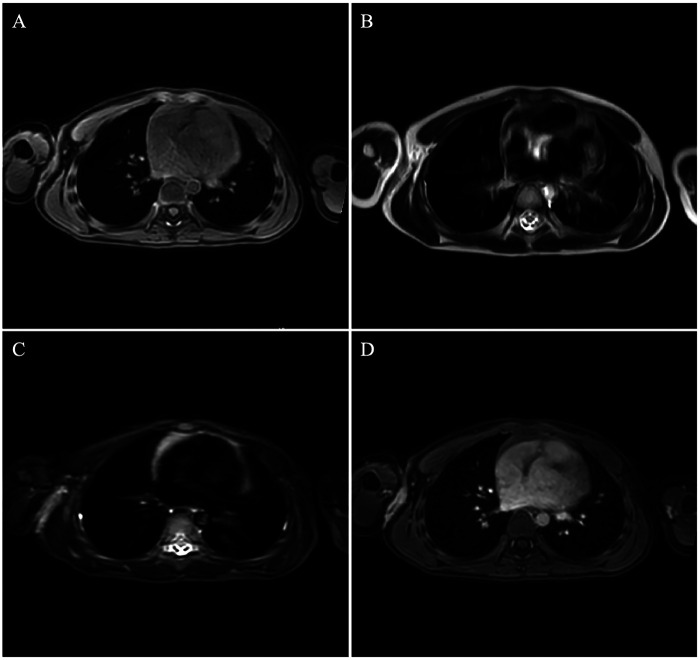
A boy with tufted angioma, aged 3 years and 10 months. TA lesion showed high, heterogeneous signal intensity on both T1WI (A) and T2WI (B) compared with muscle, while it demonstrated homogeneous, high signal intensity on fat-saturated T2WI (C). (D) Contrast-enhanced scan showing significant homogeneous enhancement. TA, tufted angioma; T1WI, T1-weighted imaging; T2WI, T2-weighted imaging.
